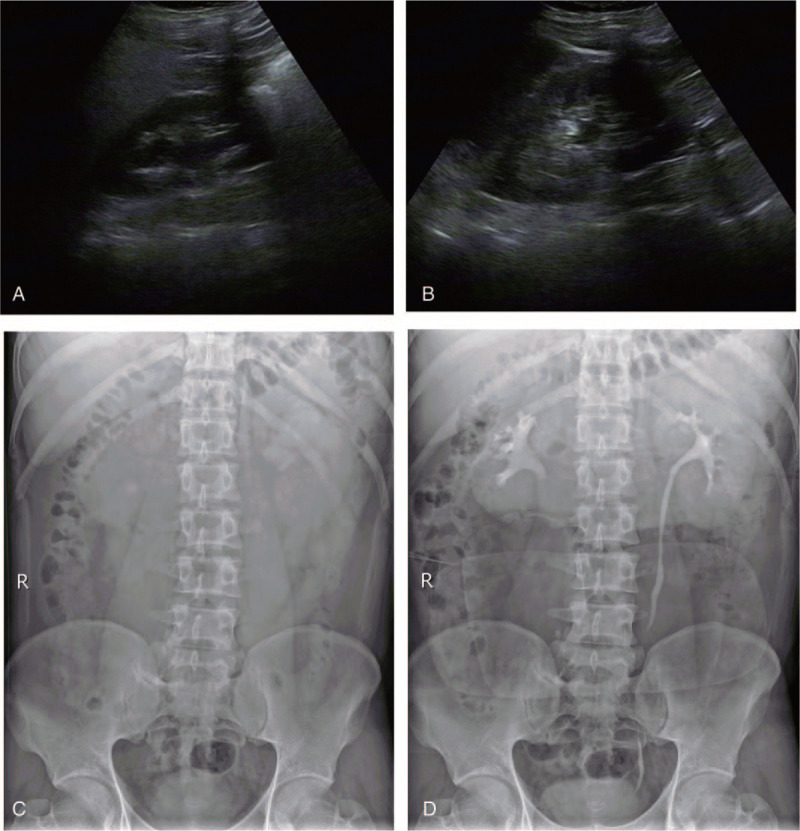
Ultrasonography of the urinary system, KUB, and intravenous urography (IVP) of patient 2. A, Right hydronephrosis. B, Right ureterectasia. C, KUB showed no positive urinary calculi in the bilateral renal area or ureteral path. D, IVP showed right hydronephrosis and dilatation in the upper and middle segments of the right ureter.
